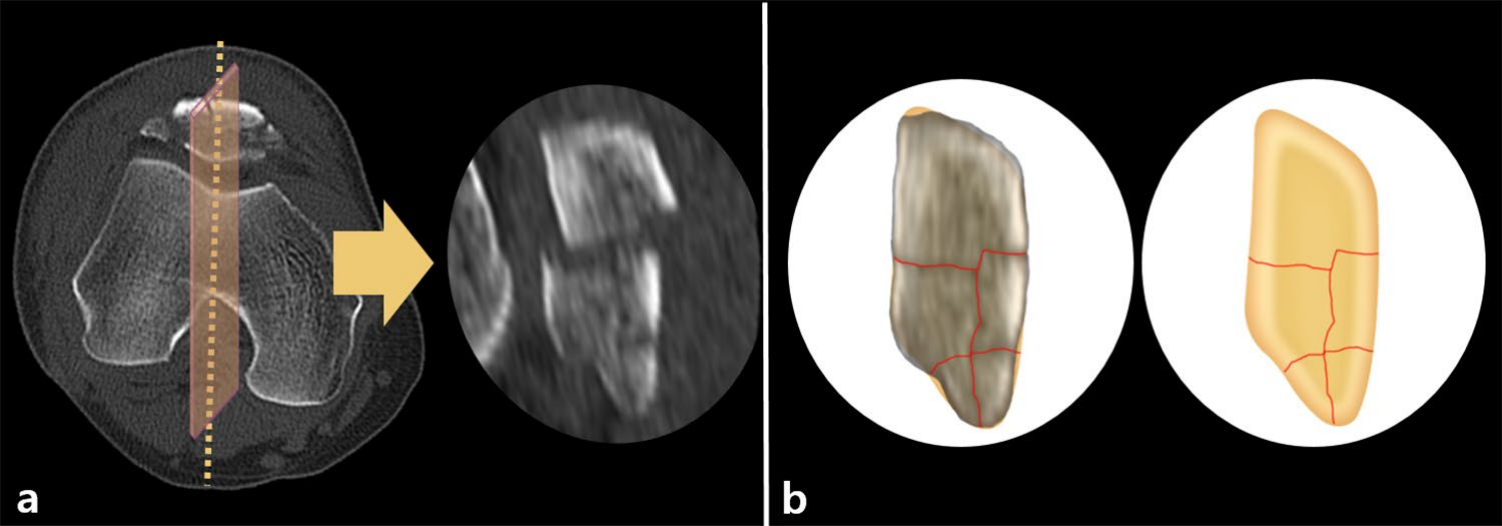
Two-dimensional computed tomography (CT) mapping of the midsagittal fracture pattern. A CT image of the patellar vertical ridge shows displaced and comminuted patellar fractures with coronal fragment and inferior pole fractures (a). The fracture fragments were reduced on the basis of a sagittal template. The fracture line (red line) was marked on the template (b).
